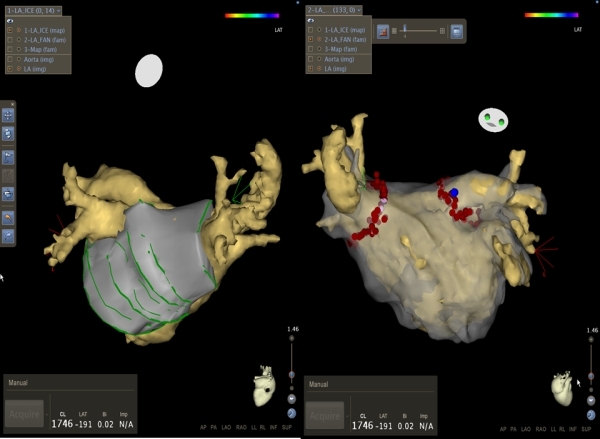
Use of intracardiac echocardiogram to facilitate image integration by CARTO 3. Left: Posterior view of the left atrium with overlay of images acquired by intracardiac echocardiogram. Right: Left atrial image following image integration with pre-acquired MRI image.
